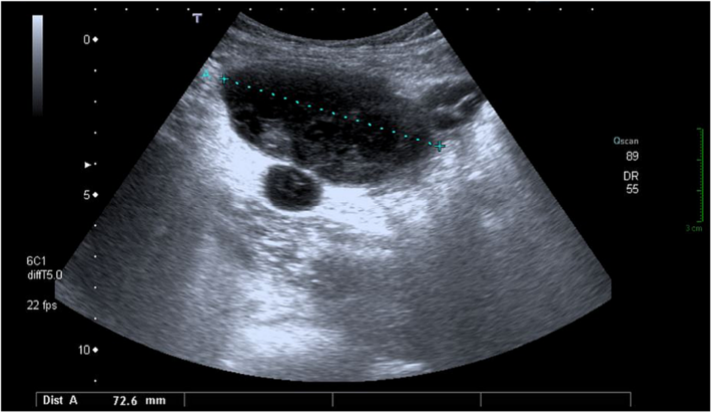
Abdominal ultrasonography. Evidence of an oval lump of 72 mm diameter and two less voluminous areas with hyperechogenic structure and hypoechoic-anechoic areas and septa in its contest
